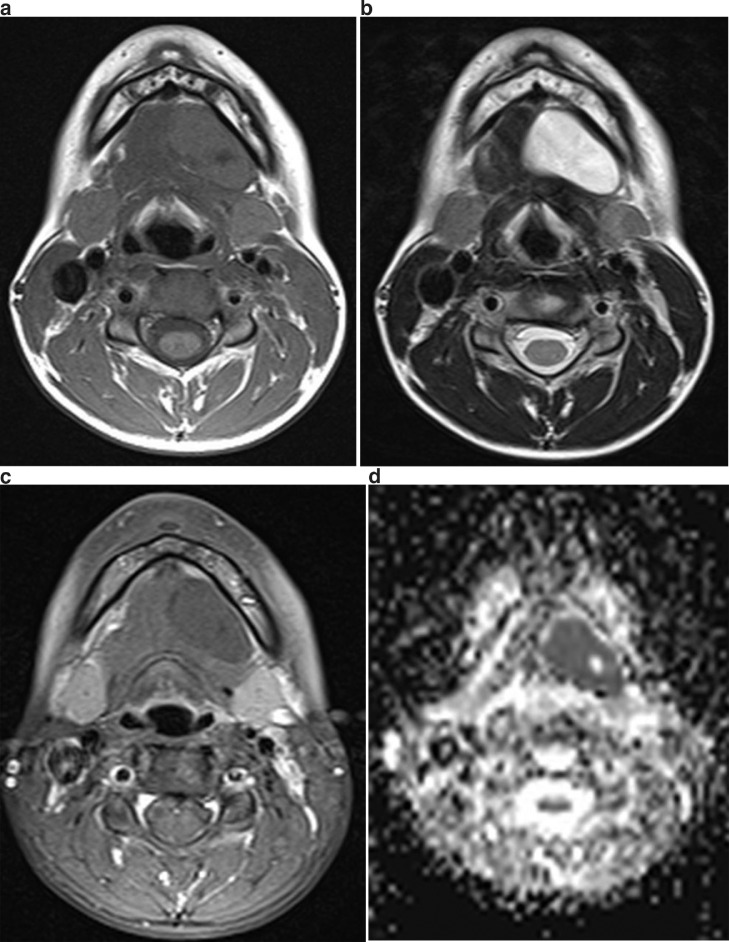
Imaging of 12-year-old female patient with dermoid cyst on left of floor of mouth. Well-circumscribed in MRI; (A) hypointense in T1WI and (B) hyperintense in T2WI; (C) no enhancement in T1W+C, (D) extremely low SI in ADC.
